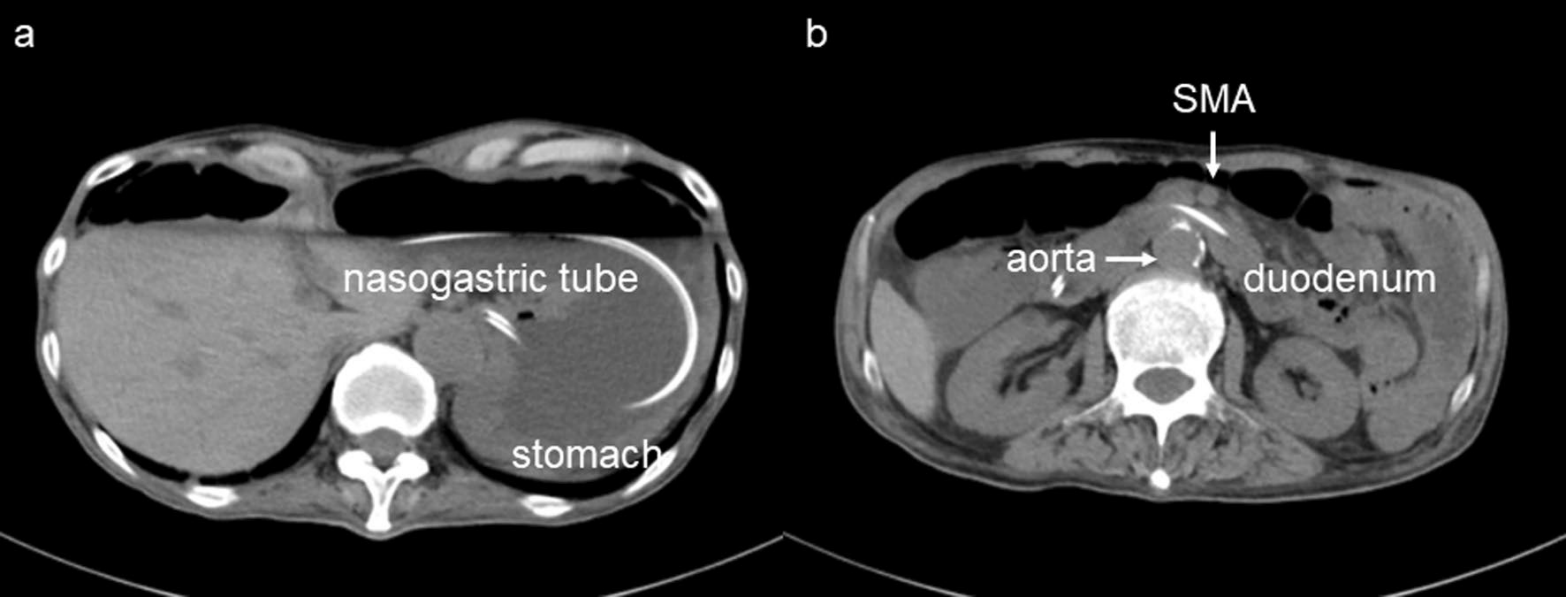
Abdominal computed tomography findings. a The stomach is dilated because of obstruction of the duodenum. b The third portion of the duodenum is compressed between the SMA and aorta
